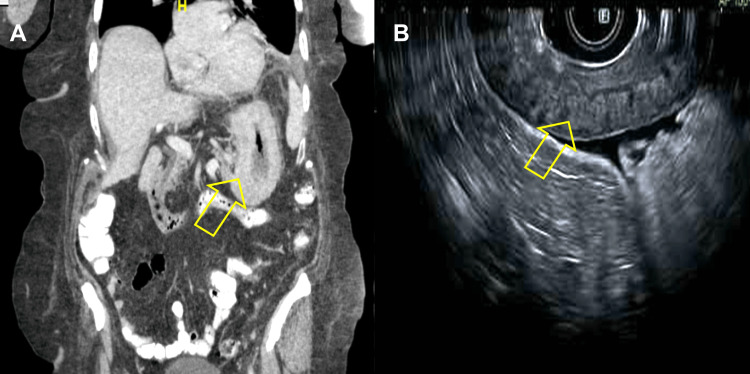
Coronal CT (A) and EUS (B) view demonstrating extensive stomach wall thickening EUS: Endoscopic ultrasound
